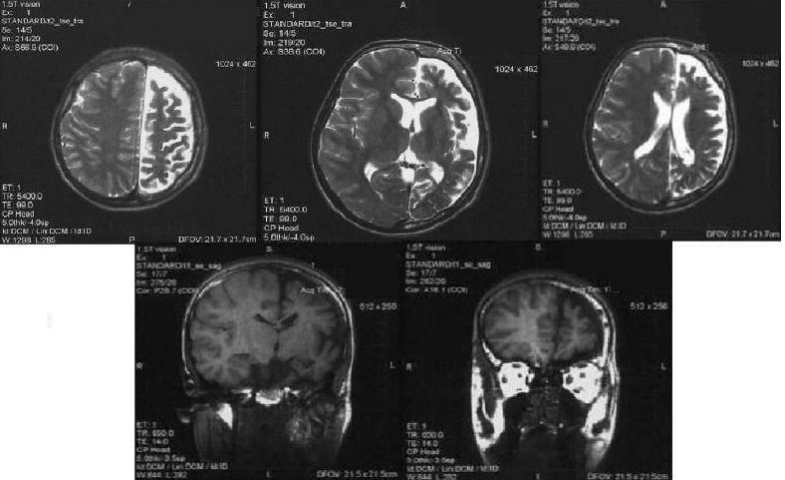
Magnetic resonance imaging of the brain, axial T2 and coronal T1 reveal atrophy of left hemisphere with signal void in sub cortical areas and wider left ventricle compared to the right one.
